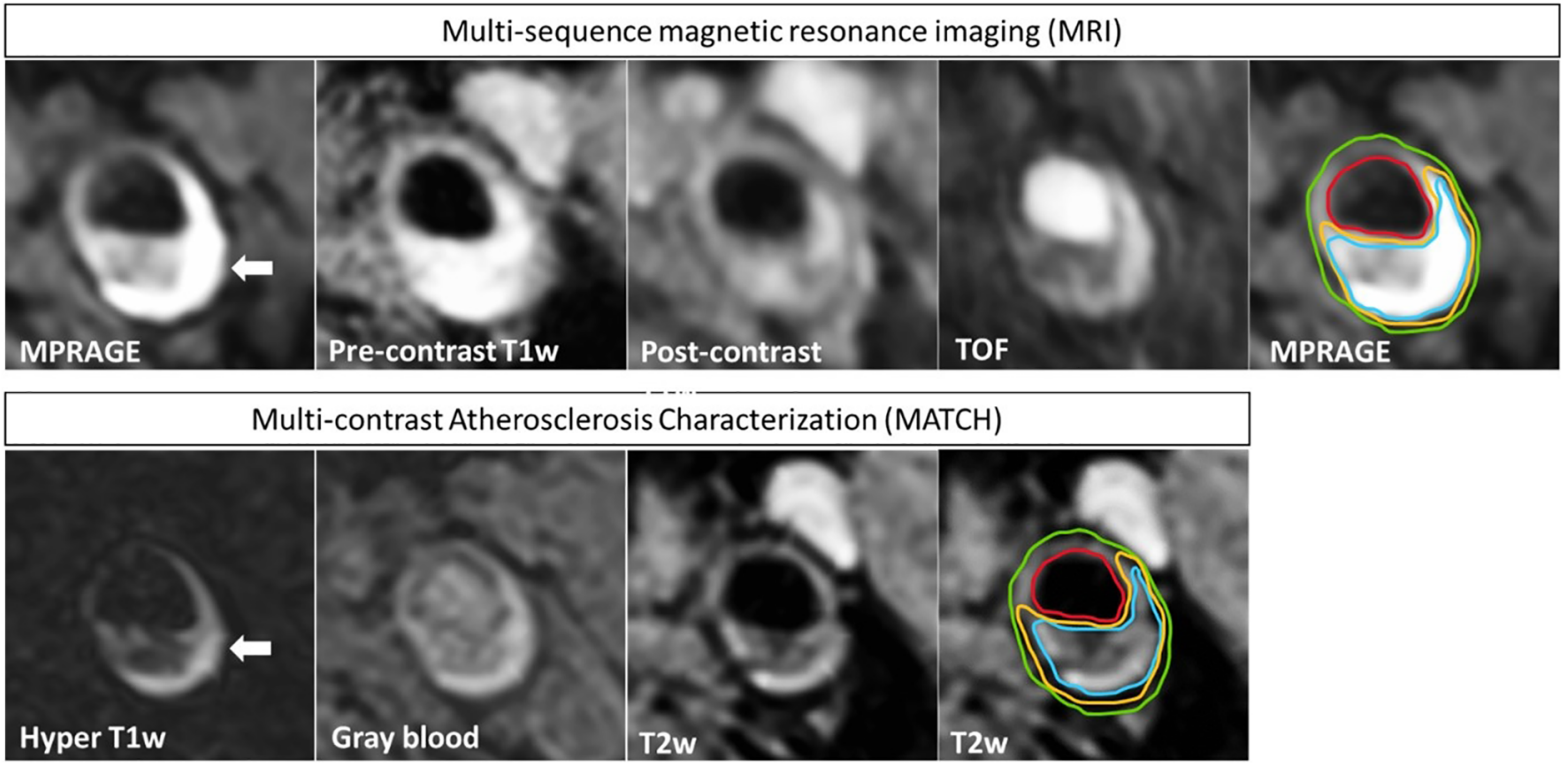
An example of a patient that exhibits an atherosclerotic plaque in the left carotid artery. The four multi-sequence MR images have been manually co-registered and are displayed in the upper row. In addition, the MATCH images with inherent co-registration are shown in the bottom row. IPH appears hyper-intense (white arrow) on hyper-T1w MATCH and MPRAGE images. Contours are shown on T2w MATCH and MPRAGE: green for the outer vessel wall, red for the lumen, yellow for LRNC, and blue for IPH. A good agreement is shown between the contours that are delineated on the MATCH vs. the multi-sequence MR images, although slight deviations can be observed.
